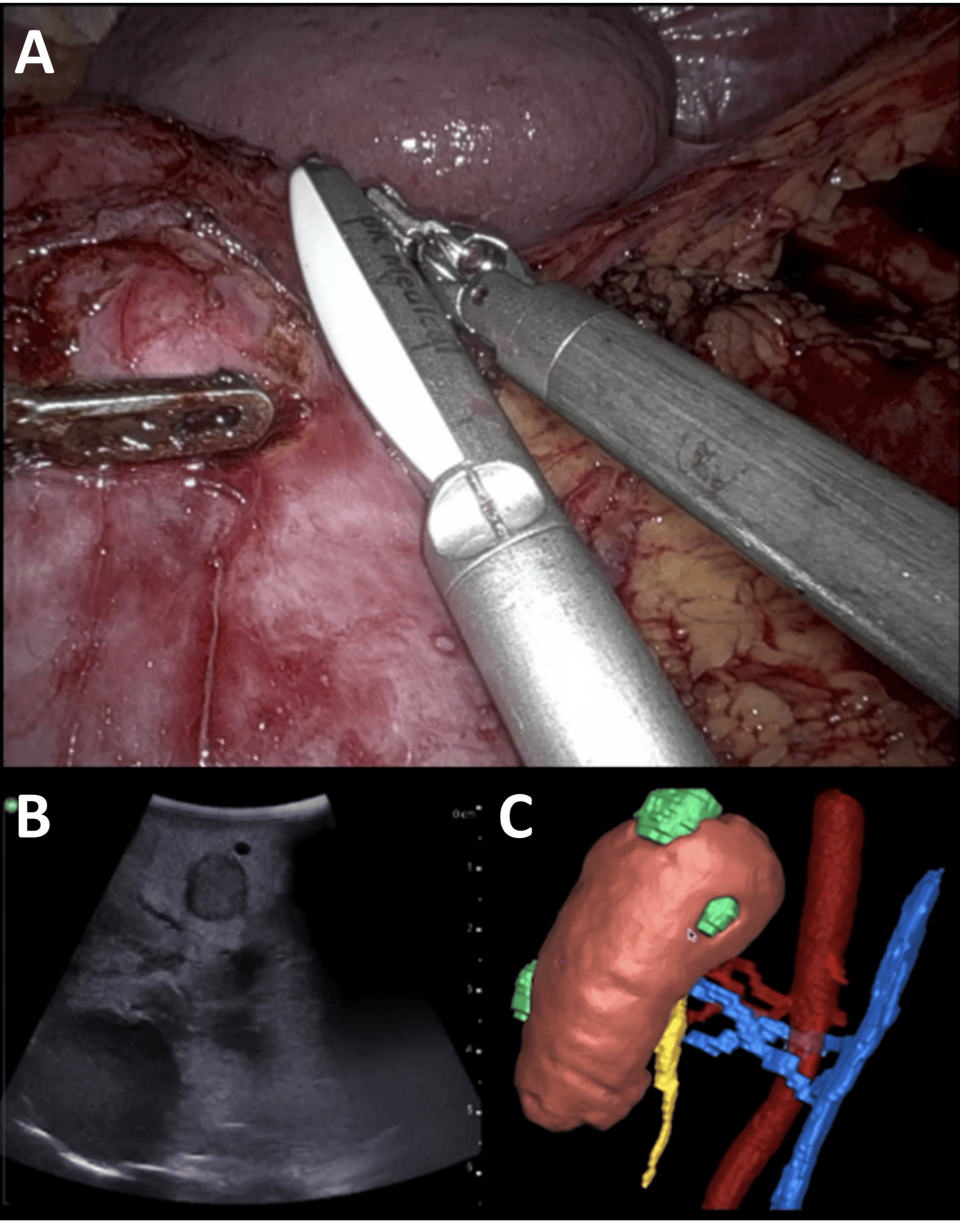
Intraoperative situs with real-time ultrasound guidance combined with the three-dimensional model. (A) Intraoperative view during robot-assisted partial nephrectomy. The needle driver is guiding the laparoscopic ultrasound probe directly onto the renal surface. (B) Laparoscopic ultrasound image depicting two of the five renal tumors. (C) Patient-specific three-dimensional kidney model reconstructed from preoperative CT imaging Image Credit: Toni Franz
